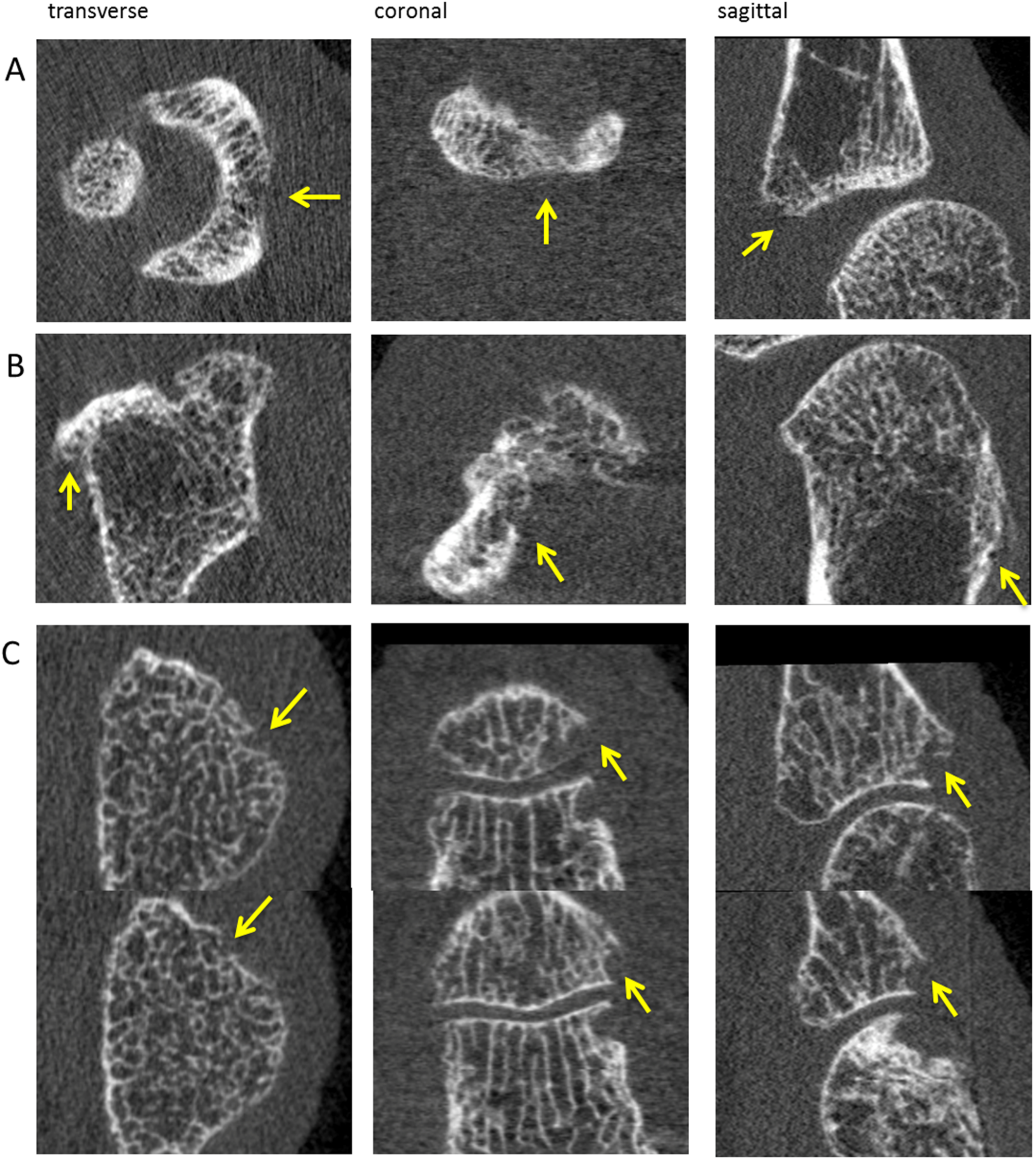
HR-pQCT images of cases leading to discrepancies between readers in scoring. Panel A: low density caused by low mineralization and/or thin cortex. Panel B: bone apposition. Panel C: multiple breaks belonging to one large break at the base of the phalanx. Abbrevations: HR-pQCT; high resolution peripheral quantitative computed tomography.
